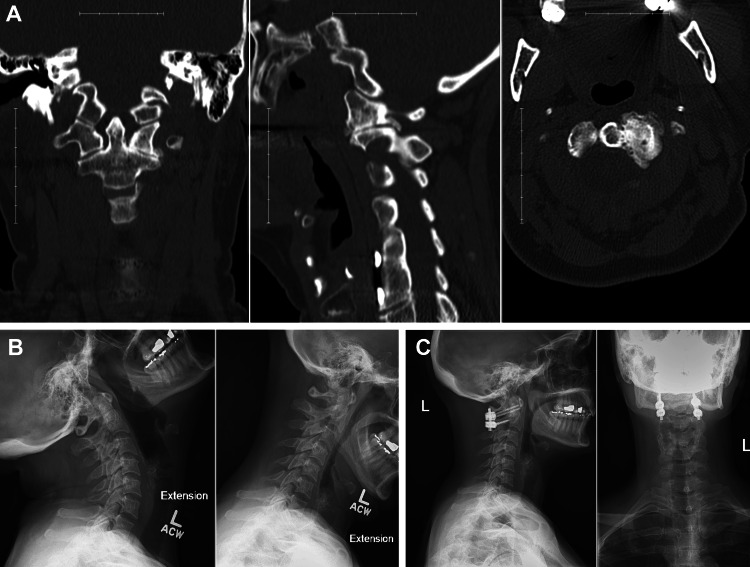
Case example of a patient in their early 50s with C1-2 spondylosis. (A) Plain computed tomographic images (left, coronal; middle, sagittal; and right, axial) of the cervical spine show greater degenerative spondylosis on the left than on the right of the C1-2 joint space. (B) Sagittal flexion-extension radiographs do not show overt instability in the cervical spine. (C) Postoperative six-week radiographs (left, sagittal; right, coronal) showing intact hardware and good alignment. *Used with permission from Barrow Neurological Institute, Phoenix, Arizona.*
